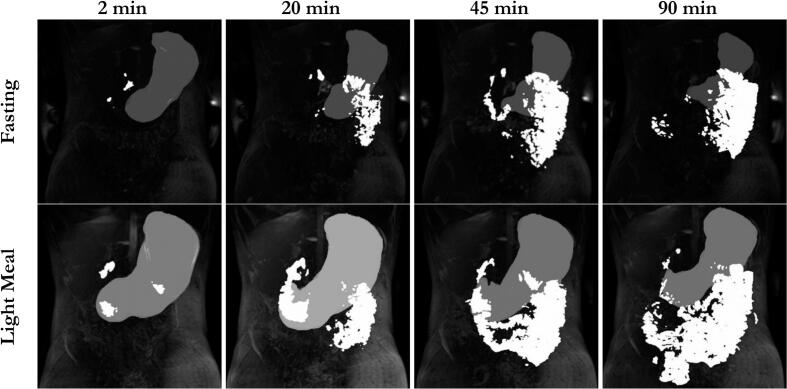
Enhanced visualization of ROIs for spreading of high signal intensity in the gastrointestinal tract in participant 8. All slices of the MRI image at the relevant time point were used for generation of the ROI, resulting in a three-dimensional object, which is shown here from the antral perspective. Transparent-grey = gastric content volume, white = areas of high signal intensity in the small intestine.
